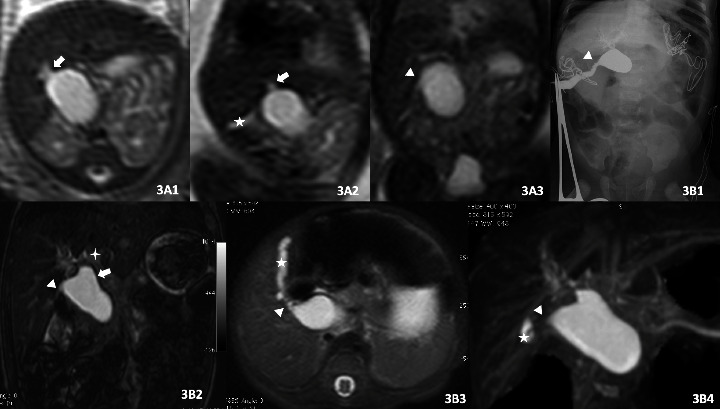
Case 8, G23 with CBA^*∗*^. A1–3: fetal MRI. Coronal (4A2–4A3) showed the cyst located in the hepatic hilar, parallel to the hepatoduodenal ligament, higher than the lower edge of the liver, and connected to the hepatic duct (white arrow) and gallbladder (white star)/duct (white triangle). Axial (4A1) showed the intrahepatic bile duct (white arrow) slight dilation. 16D postnatal MRCP^*∗*^ (B2–B4) showed that the size of cysts did not change much. The hepatic ducts were slightly dilated (white arrow), the gallbladder (white star) was stiff and caterpillar-like. Coronary T2WI (B2) showed mild edema around the portal vein (white stars). Preoperative cholangiography (B1) confirmed cystic biliary atresia. ^*∗*^CBA: cystic biliary atresia. ^*∗*^MRCP: magnetic resonance cholangiopancreatography. ^*∗*^T2WI: T2 weight imaging.
